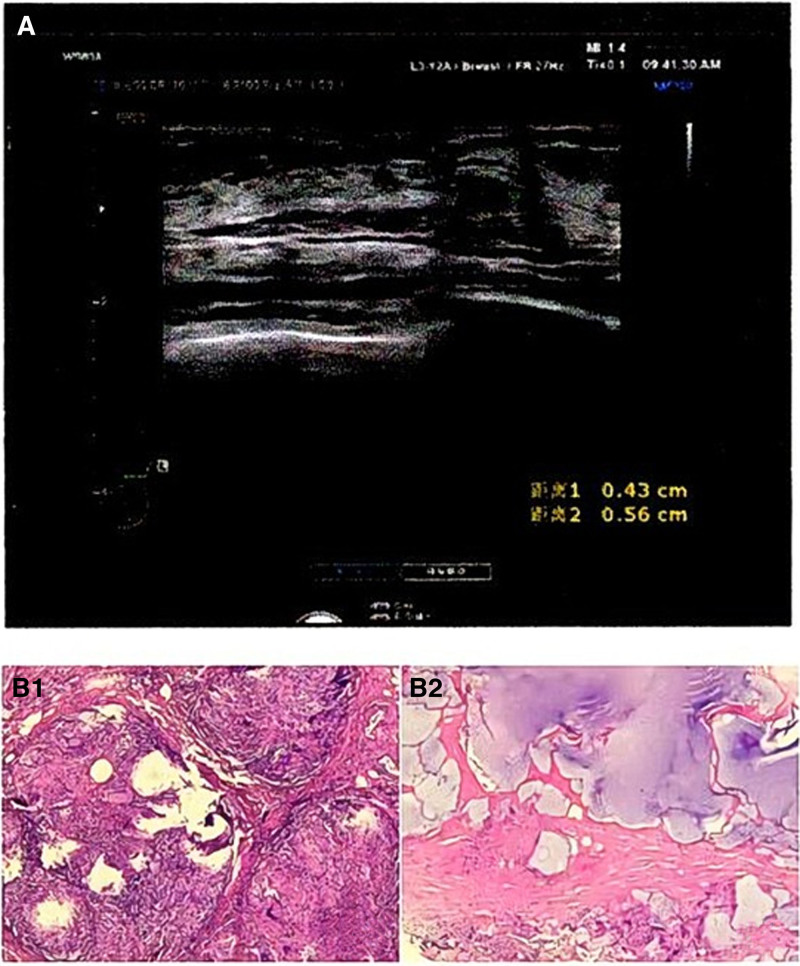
(A) B-ultrasound: (right) multiple cystic dark areas in the breast; (right) multiple hypoechoic areas in the breast and right axilla, considering the possibility of extravasation of breast augmentation fillers. (B) Pathological diagnosis: (right) breast foreign matters and foreign matter granulomas.
